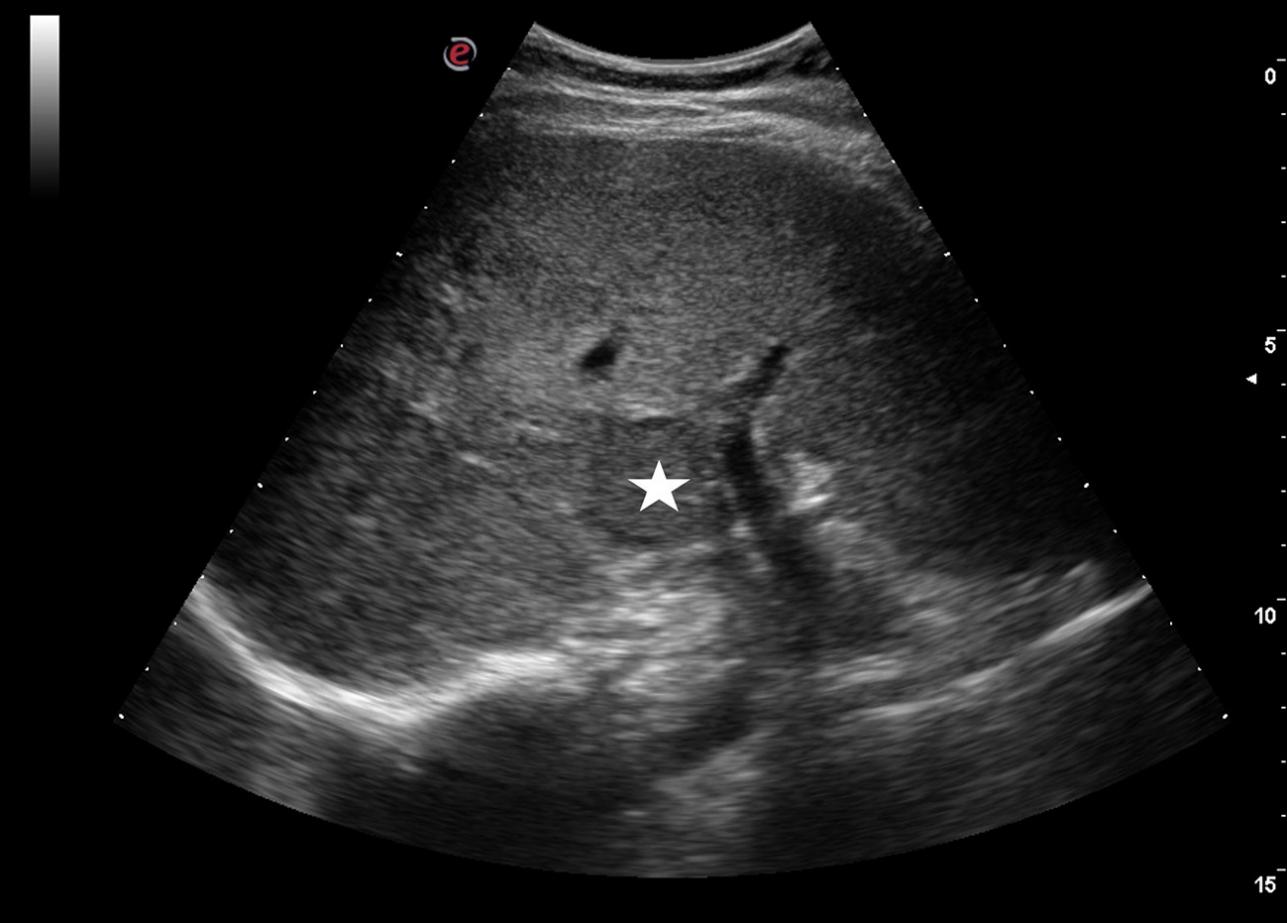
Ultrasound image of a 56-year-old female patient. A focal lesion (asterisk) is observed in the homogeneous liver parenchyma of the patient who is being followed up due to breast cancer.
